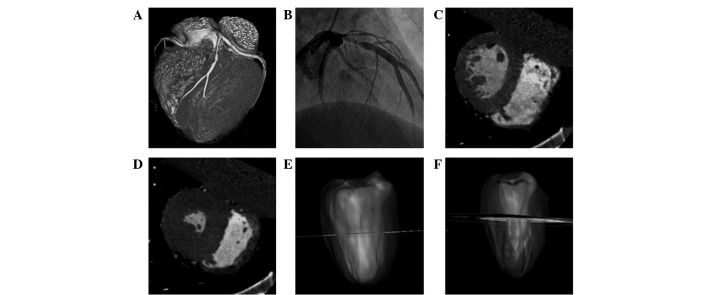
A 59-year-old male with recurrent chest stuffiness in the chest for 1 month. (A) CT-VR; (B) DSA-CAG, a moderate stenosis in the proximal end of the LAD, showing the consistency between the two approaches; (C) short-axis position of the left ventricle at the end of diastasis; (D) short-axis position of the left ventricle at the end of systole; (E) VR of the ventricular volume at the end of diastasis based on cardiac function analysis; (F) VR of ventricular volume at the end of systole. CT-VR, computed tomography-volume render; DSA-CAG, digital subtraction angiography-coronary angiography; LAD, left anterior descending artery; VR, volume render.
